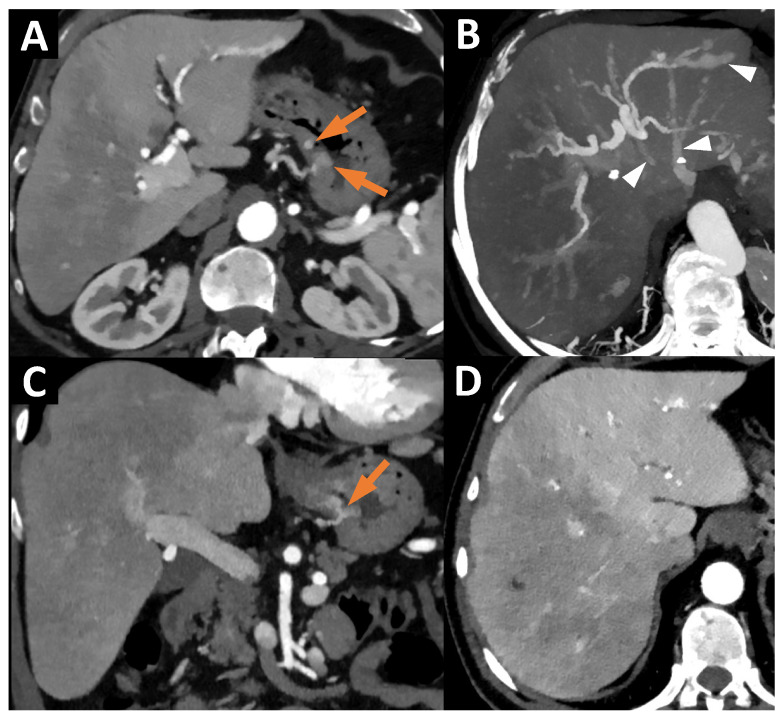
(A) Axial CT at the arterial phase (40 keV reconstruction), showing telangiectases of the stomach body (orange arrows). (B) Axial CT at the arterial phase, with Maximum Intensity Projection (MIP) 25 mm, showing a voluminous arteriovenous shunt with early enhancement of the left hepatic vein (white arrowheads). (C) Coronal CT at the arterial phase (40 keV reconstruction), showing telangiectases of the stomach body (orange arrow). (D) Axial CT at the arterial phase (40 keV reconstruction), showing heterogeneous hepatic enhancement linked to the arteriovenous shunt between the left branch of the hepatic artery and the left hepatic vein.
